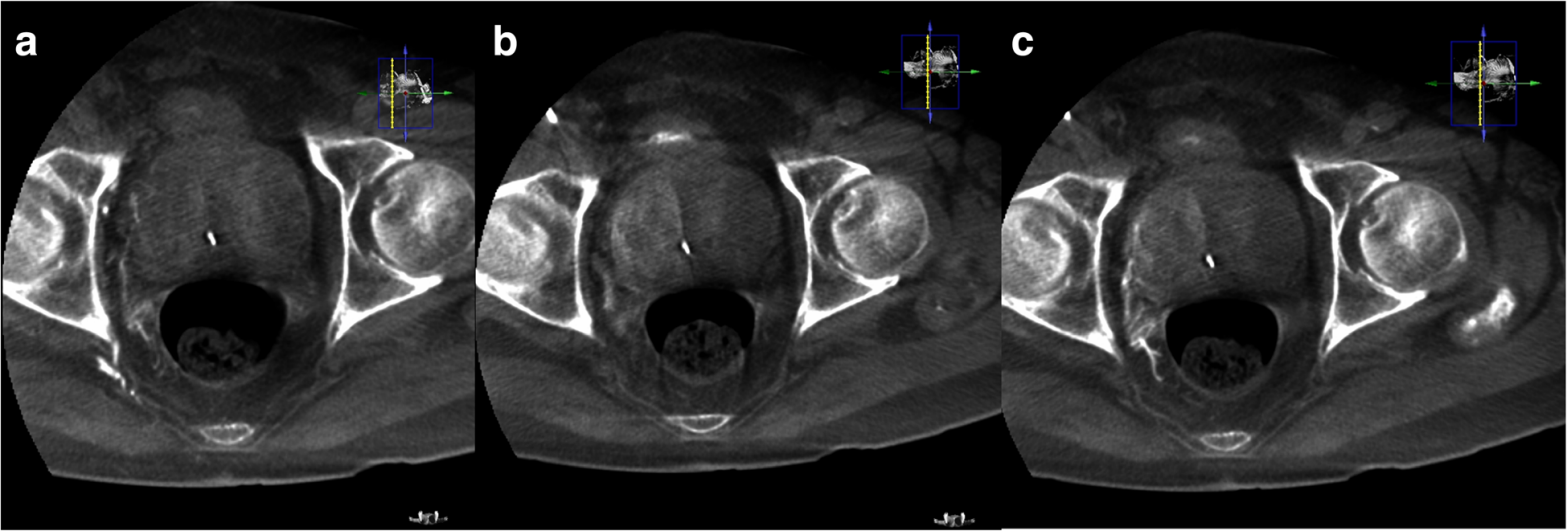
a Contrast CBCT of the RPA shows small distal branches supplying the right aspect of the rectum. Decreased contrast medium retention in the left aspect of the prostate gland can be seen, as compared with Fig. 3(a). b Non-contrast CBCT after embolization of the RPA shows retention of contrast medium in the right aspect of the prostatic parenchyma and the distal branches, without filling defect. c Contrast CBCT after embolization of the RPA shows no further enhancement of the right aspect of the prostate gland
